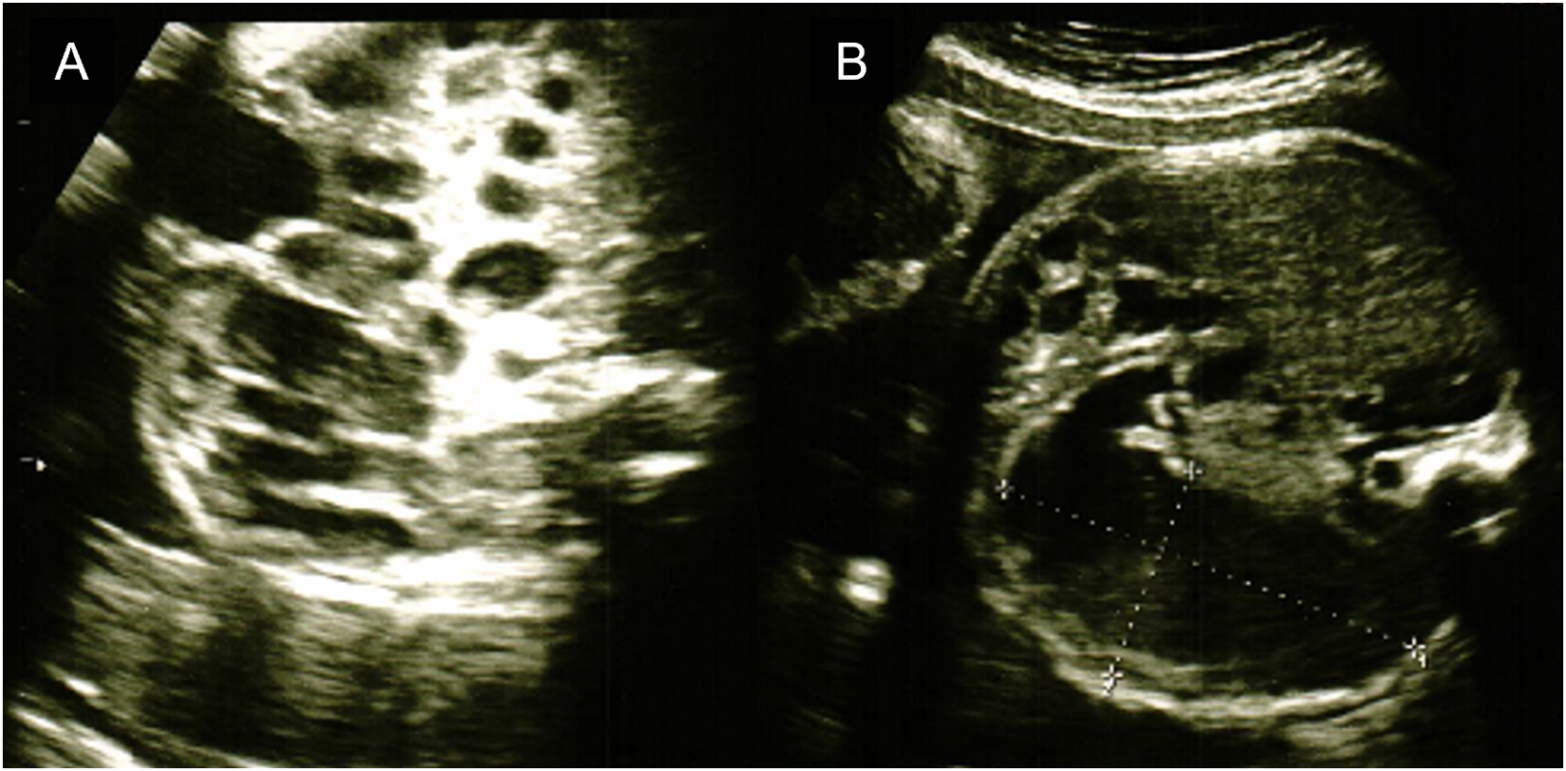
The fetal ultrasonography. (A) Image of honeycomb-shaped intestinal dilation. (B) Image of gastric bubble dilation.
